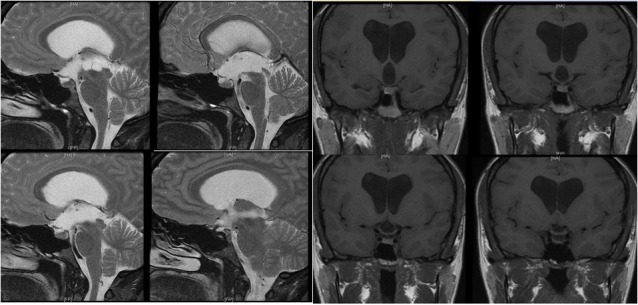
Patient imaging at presentation (Left) T2 weighted sagittal brain MRI demonstrating an enlarged multi-loculated cystic structure located in the third ventricle with worsened ventriculomegaly. (Right) T1 weighted non-contrast coronal brain MRI demonstrating an enlarged multi-loculated cystic structure located in the third ventricle with worsened ventriculomegaly.
